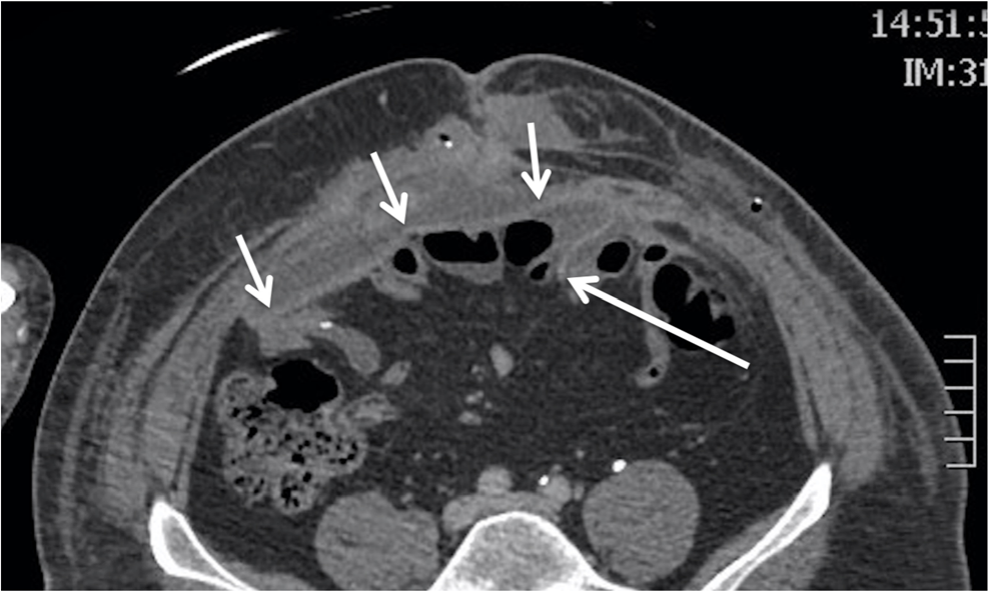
Postoperative axial CT showing an intraperitoneal mesh (*short white arrows*). The mesh has become detached (*white long arrow*), with the left lateral edge coming to lie several centimetres deep to the anterior abdominal wall. This offers the opportunity for recurrence at this site and may also cause deep adhesions
